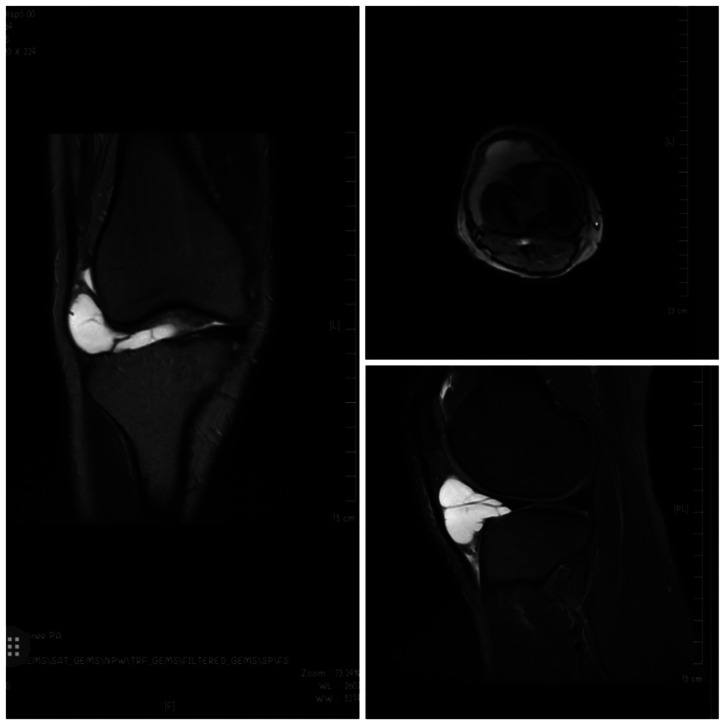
This image represent the MRI findings of the individual.
